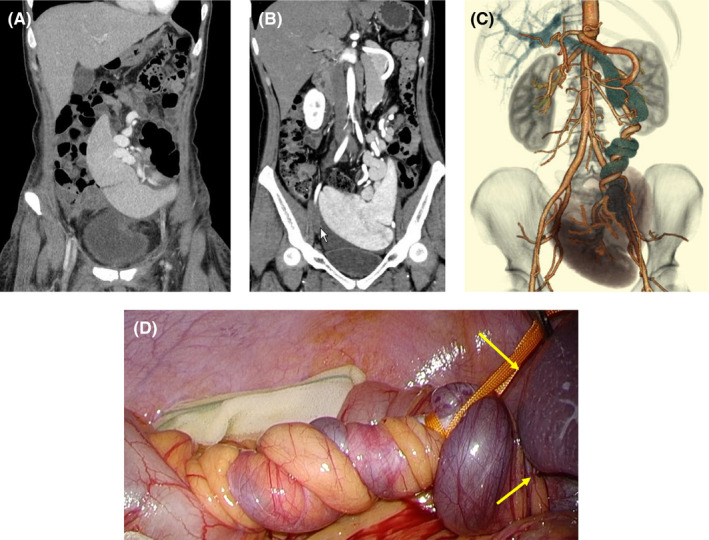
(A) CE‐CT after delivery. The spleen was located in the middle of the abdomen. (B) CE‐CT a few months post‐delivery. The spleen had become enlarged and it had also moved into the pelvis. (C) 3D construct CT. The splenic artery and vein were spirally twisted by 1260°. (D) Laparoscopic image. Yellow arrows (→) indicate the spleen. The splenic artery and vein were both spirally twisted
